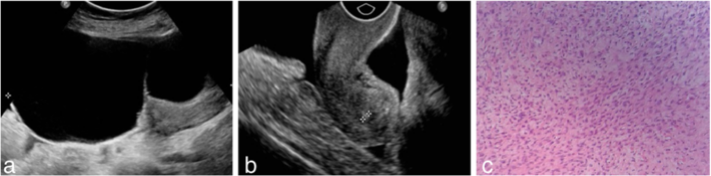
A 63-year-old woman with pelvic mass was found by physical examination. a A 133 mm*69 mm*169 mm well-circumscribed mixed mass was detected in the right ovary by ultrasound examination; b ultrasound detected ascites in the pelvis; c pathological findings showed ovarian thecofibroma with collagen change
